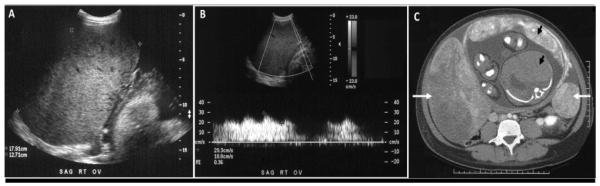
A.* Transbdominal ultrasound image of the right ovary showing an enlarged heterogeneous complex mass measuring 13.0×18.7×11.0cm with moderate ascites. *B.* Doppler ultrasound of the right ovarian mass at 35 weeks gestation showing abnormal Doppler signals, with a peak systolic velocity of 34.5cm per second, end diastolic velocity of 21.7cm per second, and a resistive index of 0.37, suggestive of neovascularization and decreased impedance to blood flow. *C.* Contrast-enhanced computed tomography scan of the abdomen at 35 weeks gestation showing bilateral ovarian masses (white arrows) and a cross section of the uterus, placenta, and fetus (black arrows).
